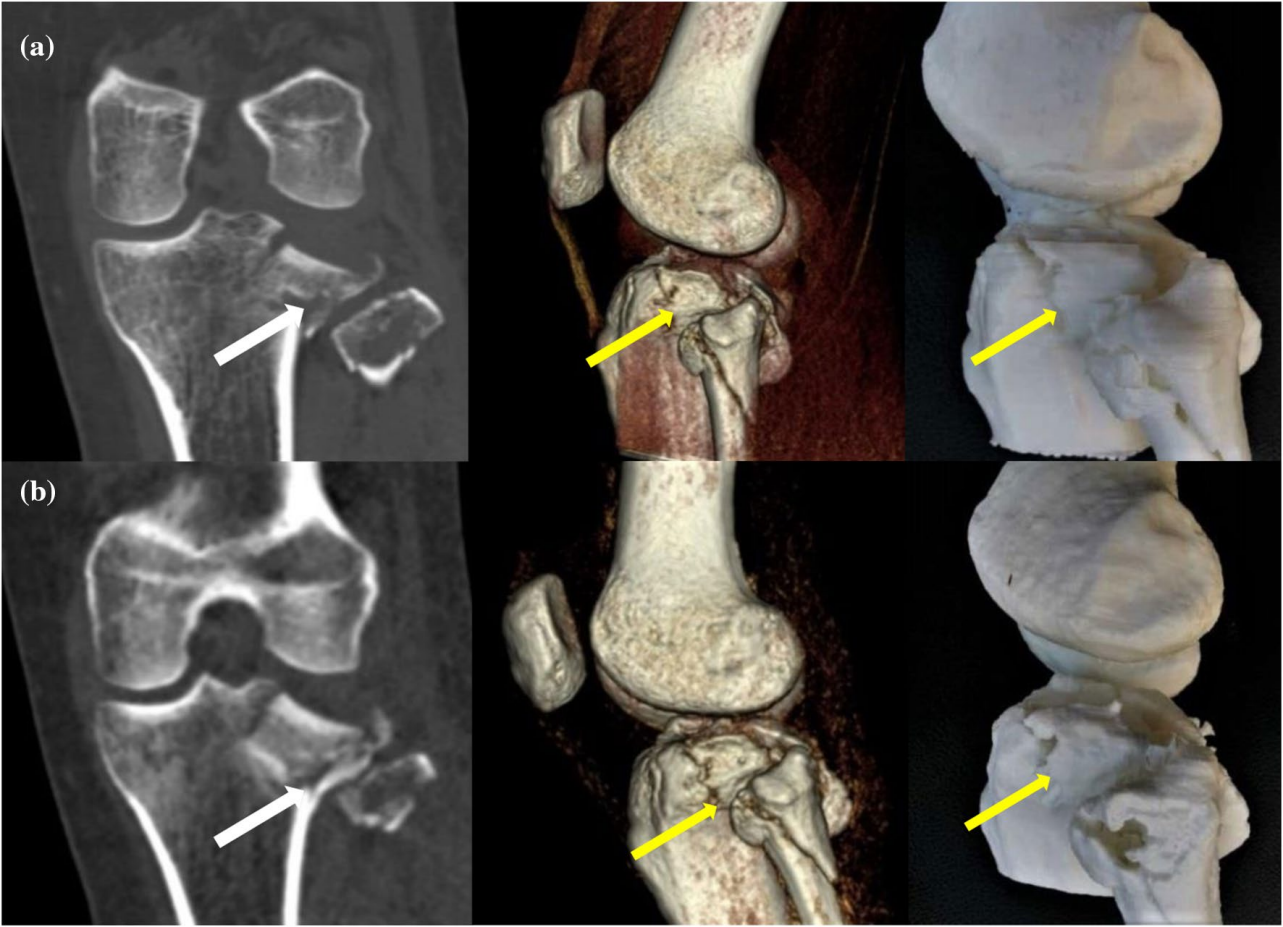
Representative case study 2. A 36-year-old woman with comminuted fracture of tibial plateau and fibula underwent two scans (at the first visit and 8 days later). The standard-dose (a) and ultra-low-dose (b) protocols were used to generate three-dimensional printing models from 3D CT data. The direction of the image quality (white arrows) and locations of the fragments (yellow arrows) are identifiable with both scans, meeting the needs of clinical diagnosis and treatment. The 3DP score was 3 points. The surface of the bone models is regular and flat, which is suitable for preoperative evaluation
